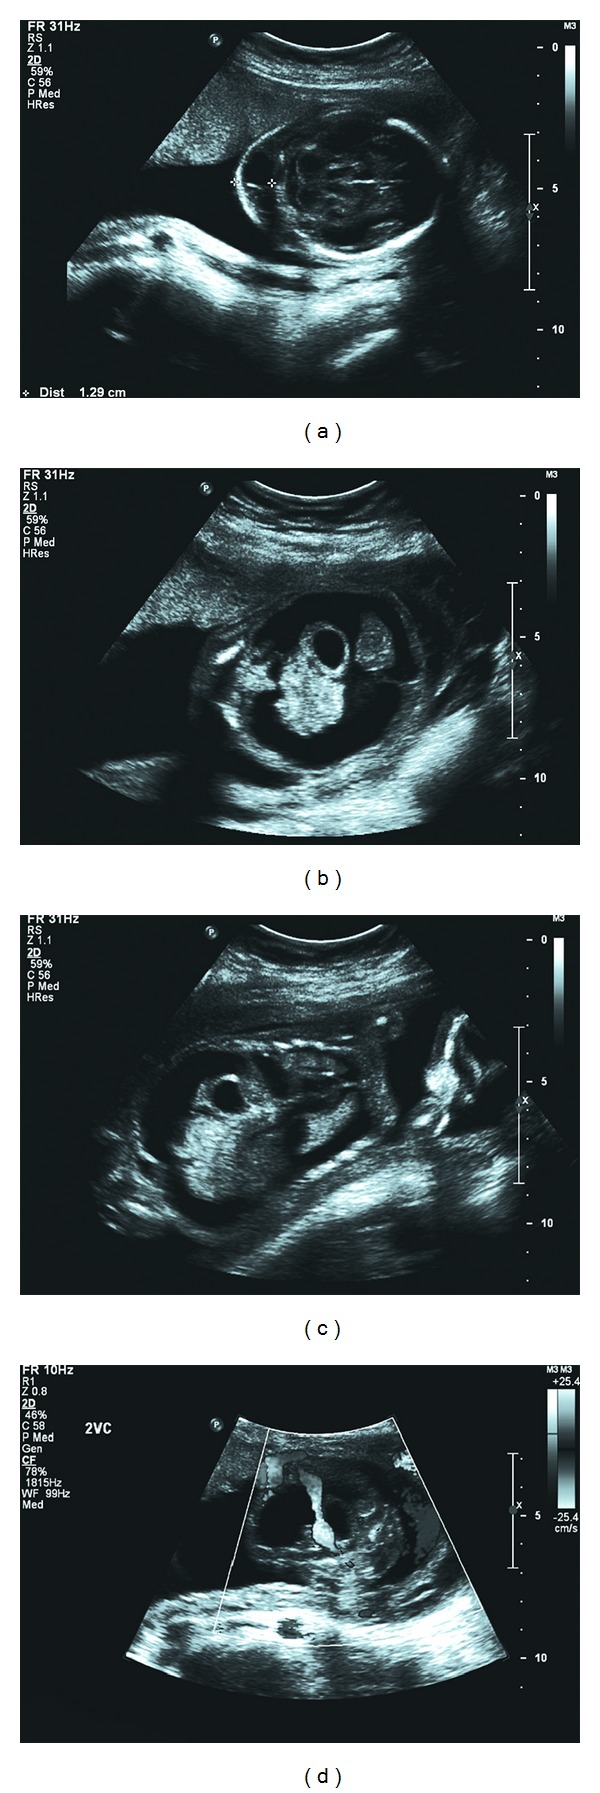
Ultrasound images of (a) septated cystic hygroma, (b) ascites with free floating stomach and small intestine, (c) ascites and pleural effusions (coronal view), and (d) single umbilical artery.
